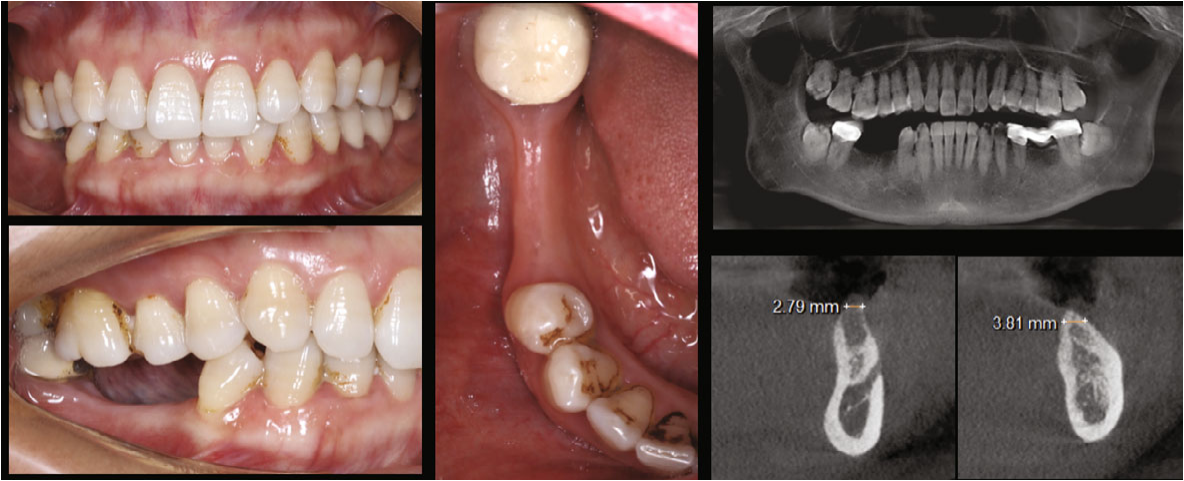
Clinical and radiographic assessment of the patient at baseline. Notice the limited horizontal bone availability for the placement of implants in positions 4.5 and 4.6.
